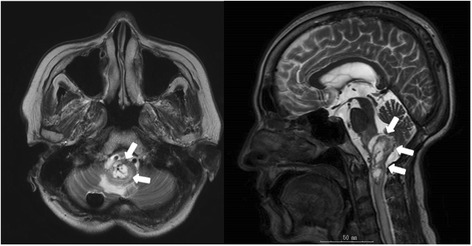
Head MRI. The MRI scan reveals an a brainstem hemangioblastoma
